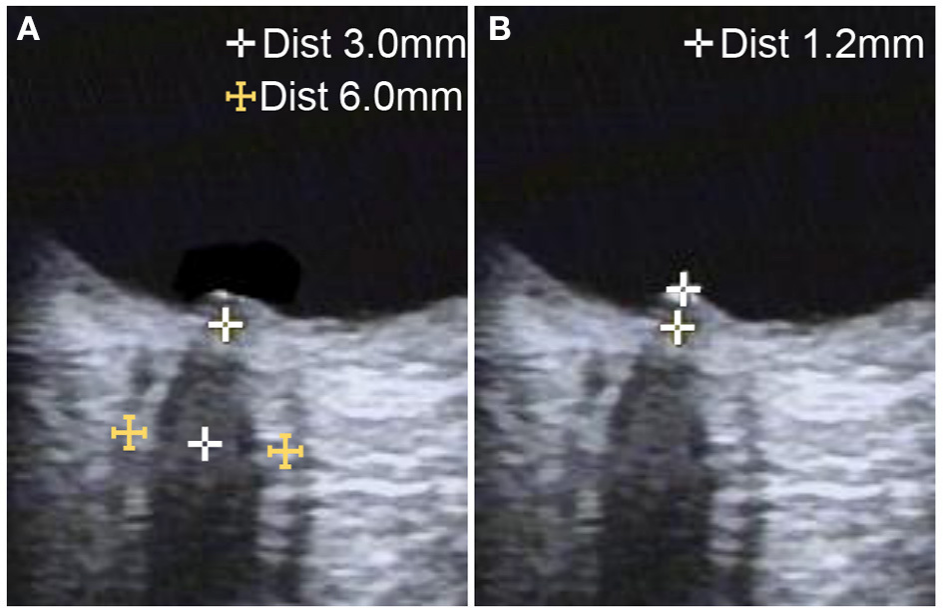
Ultrasonographic B-mode images of optic nerve measurement in a patient with idiopathic intracranial hypertension. (A) The optic nerve sheath diameter was measured 3 mm behind the papilla on the axial plane, showing the optic nerve in its longitudinal course. (B) Optic disc elevation was measured between the fundus and the dome of the papilla.
